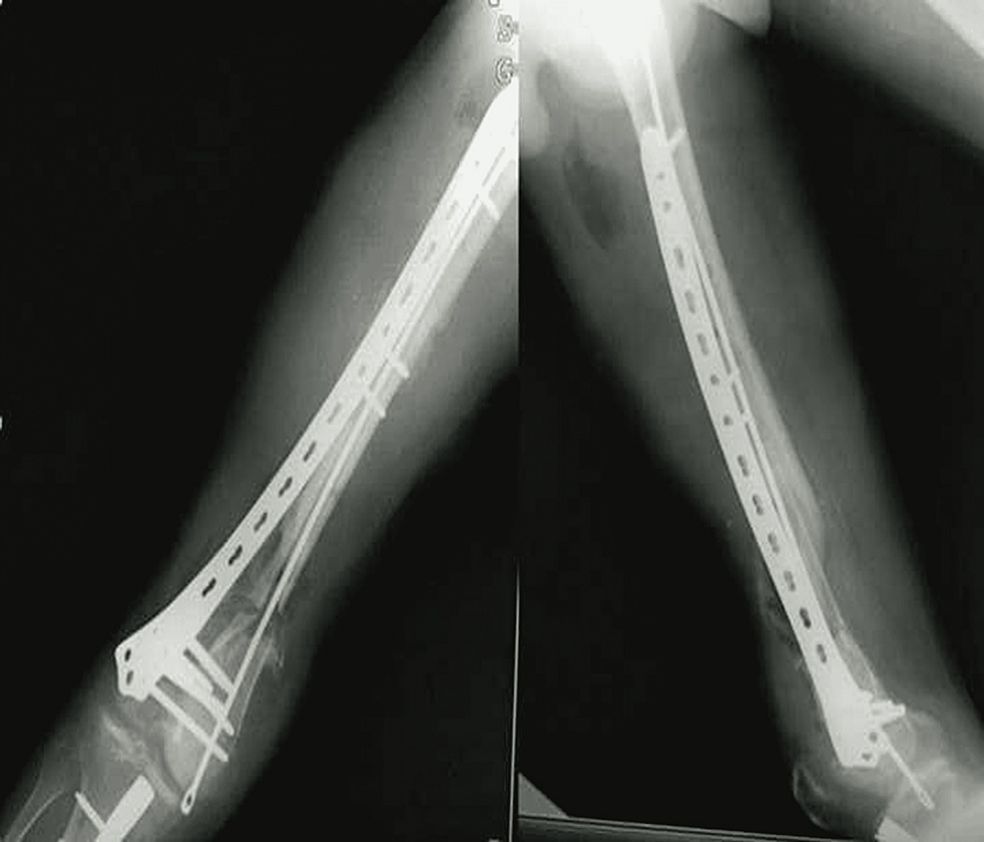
X-ray right femur showing internal fixation with distal femur locking plate and a medially placed ender nail in situ
